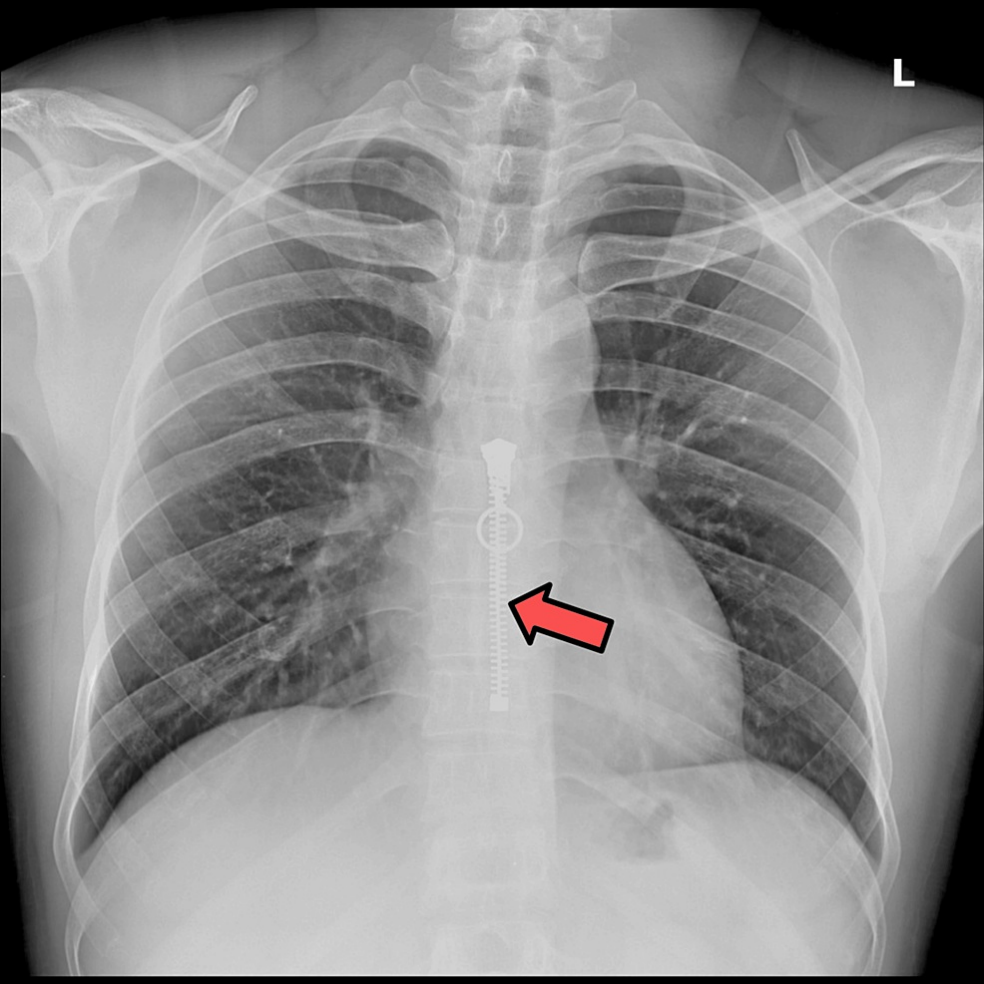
A 31-year-old male with a chest radiograph affected by clothing metallic material (arrow pointing to a zipper).
